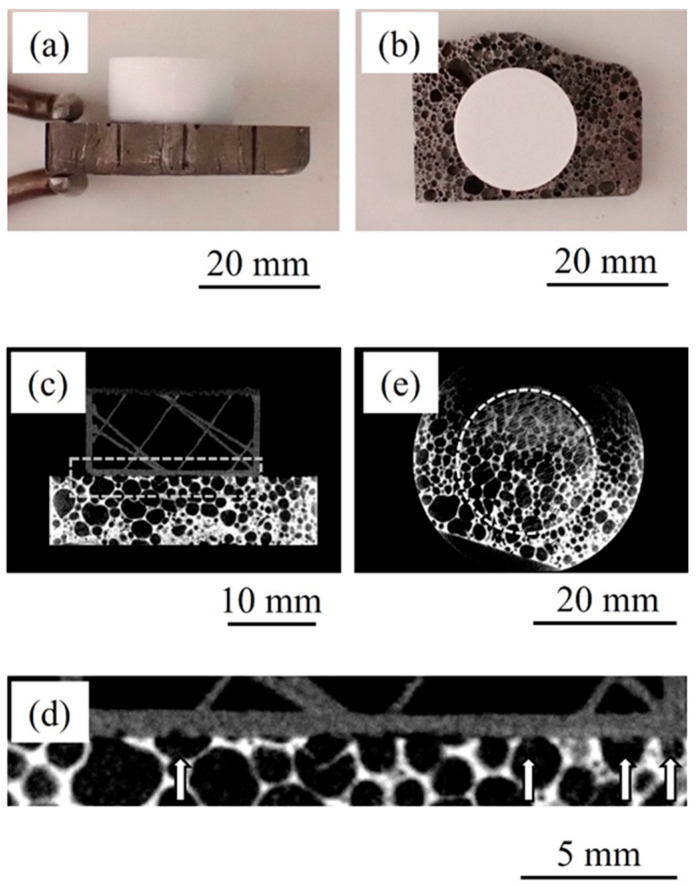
Bonded sample in which resin was directly printed on unheated aluminum foam. (a) Side view. (b) Top view. (c) X-ray CT image viewed from side. (d) Enlarged image of dotted box in (c). White arrows show where the resin penetrated into pores. (e) X-ray CT image viewed from above.
